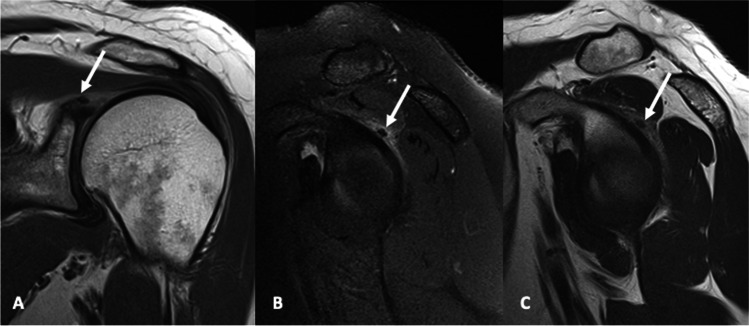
A 39-year-old male presented with a sudden onset of left shoulder pain. A Coronal proton density, B sagittal T2 with fat saturation and C sagittal T2-weighted images demonstrating capsular calcification involving the posterosuperior glenohumeral joint (arrow) with adjacent pericapsular edema
